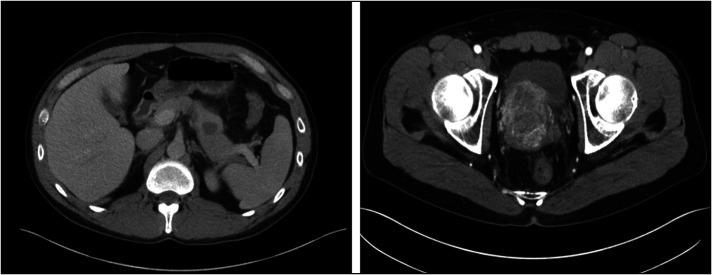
Abdominal and pelvic computed tomography showing a 4 cm by 5.1 cm pelvic mass protruding into the bladder (right) and a 2.2 cm hypodense lesion within the pancreatic body (left).
